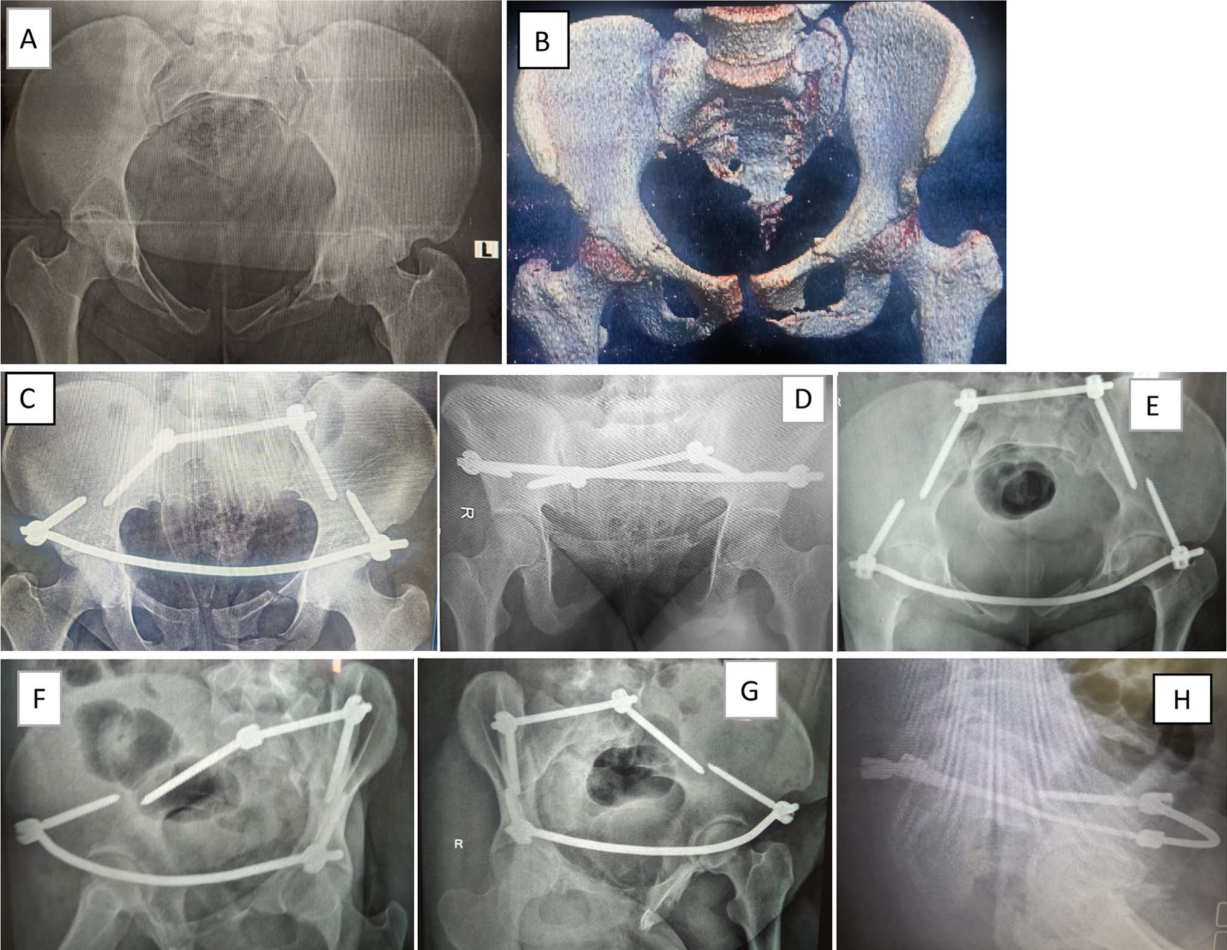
A, B Preoperative radiograph showing left fracture sacrum Denis type 2 in 21 years old male. C–H Final follow-up different radiographs showing iliac screws accurate trajectory
